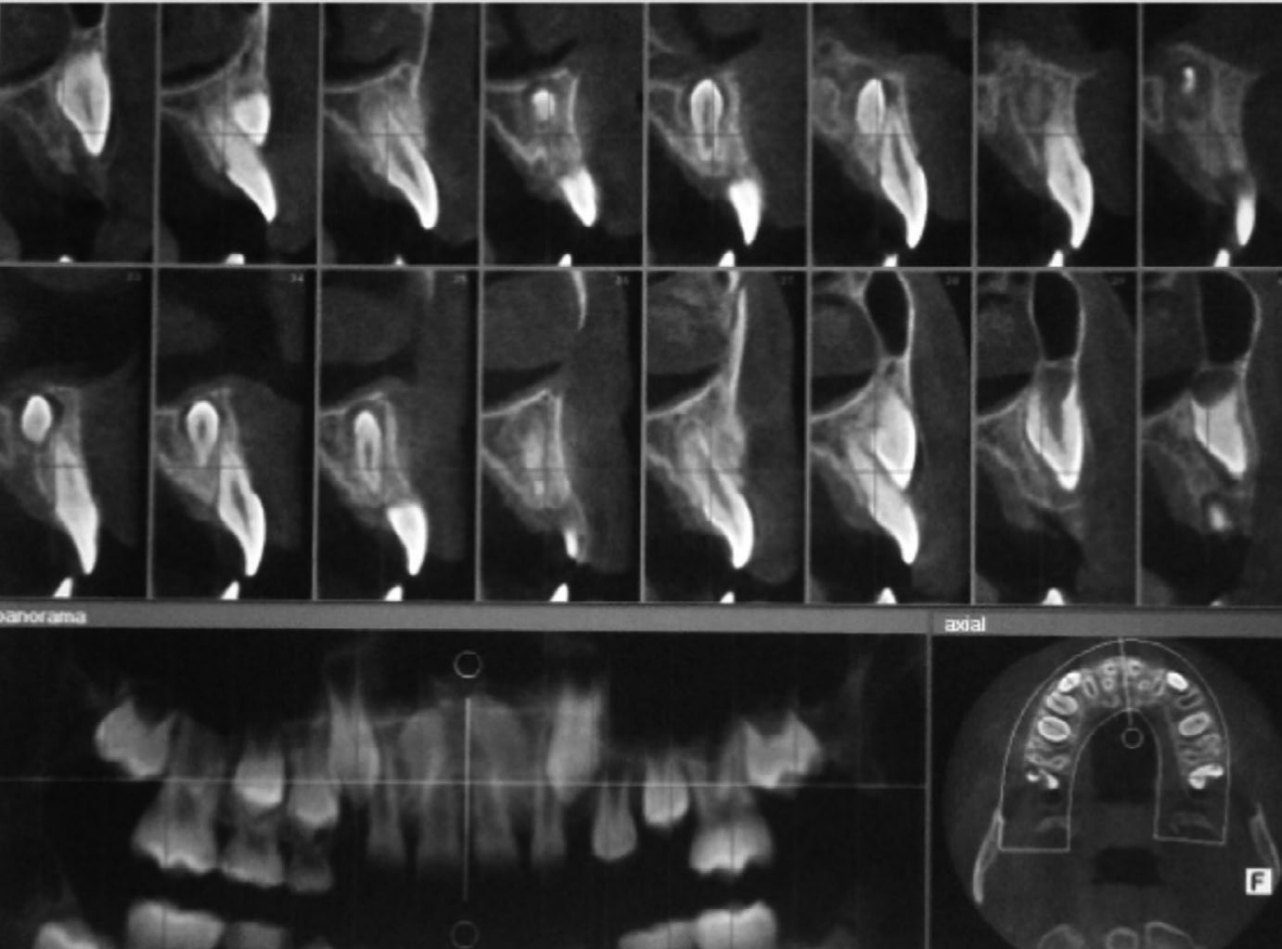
Sagittal CBCT images showing deeply inverted position of both mesiodens and their proximity to the adjacent root of permanent incisors
